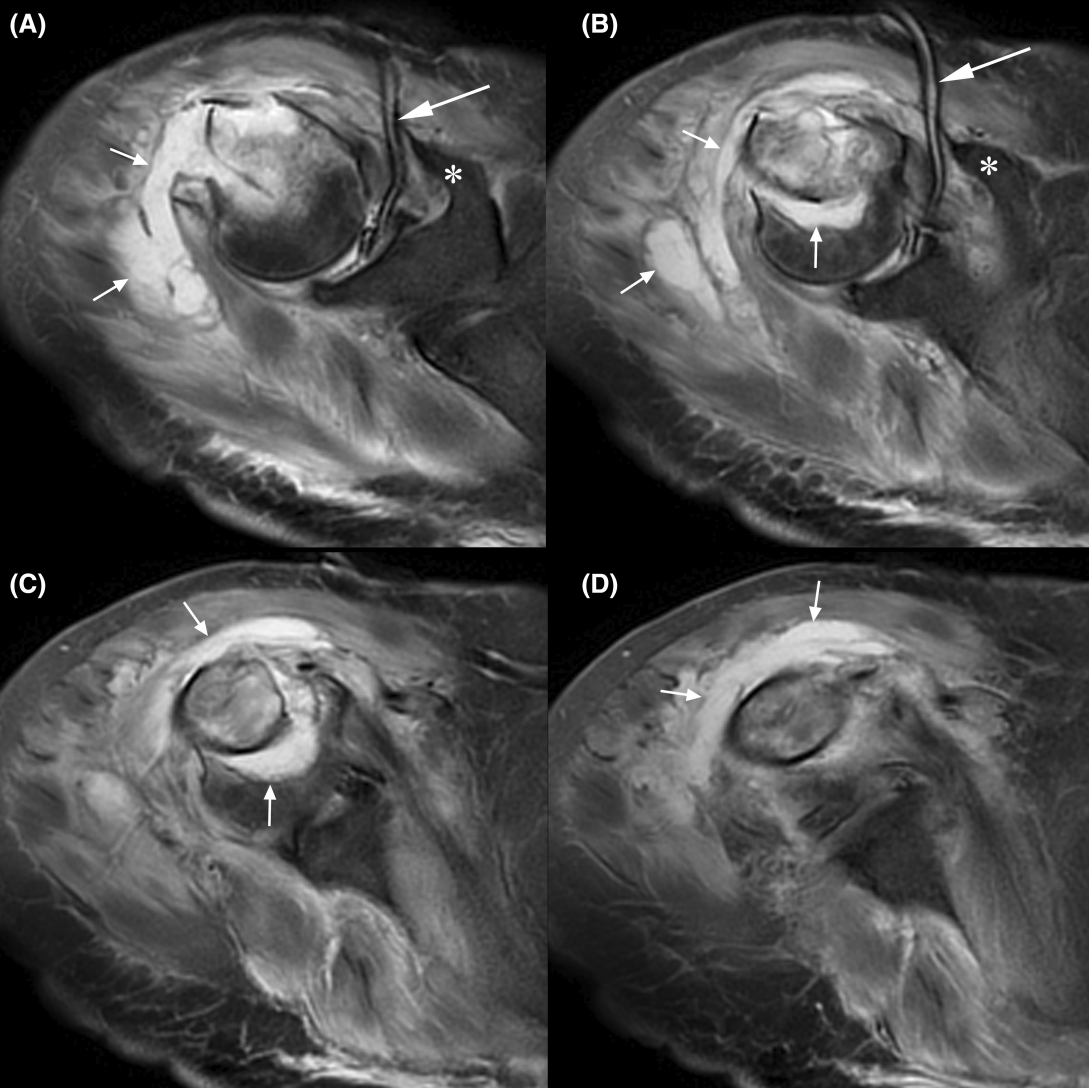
Sequential T2 (fluid enhancing) MR images in coronal view (A is most proximal, D is most distal). Asterisks (*) indicate the coracoid process, longer arrows indicate a Jackson–Pratt drain within the glenohumeral joint (A, B), and shorter arrows indicate the abscess. The abscess clearly extends into the medullary region of the bone at the fracture site (e.g., vertical arrows in B and C). The scan was obtained 13 days after the fracture.
